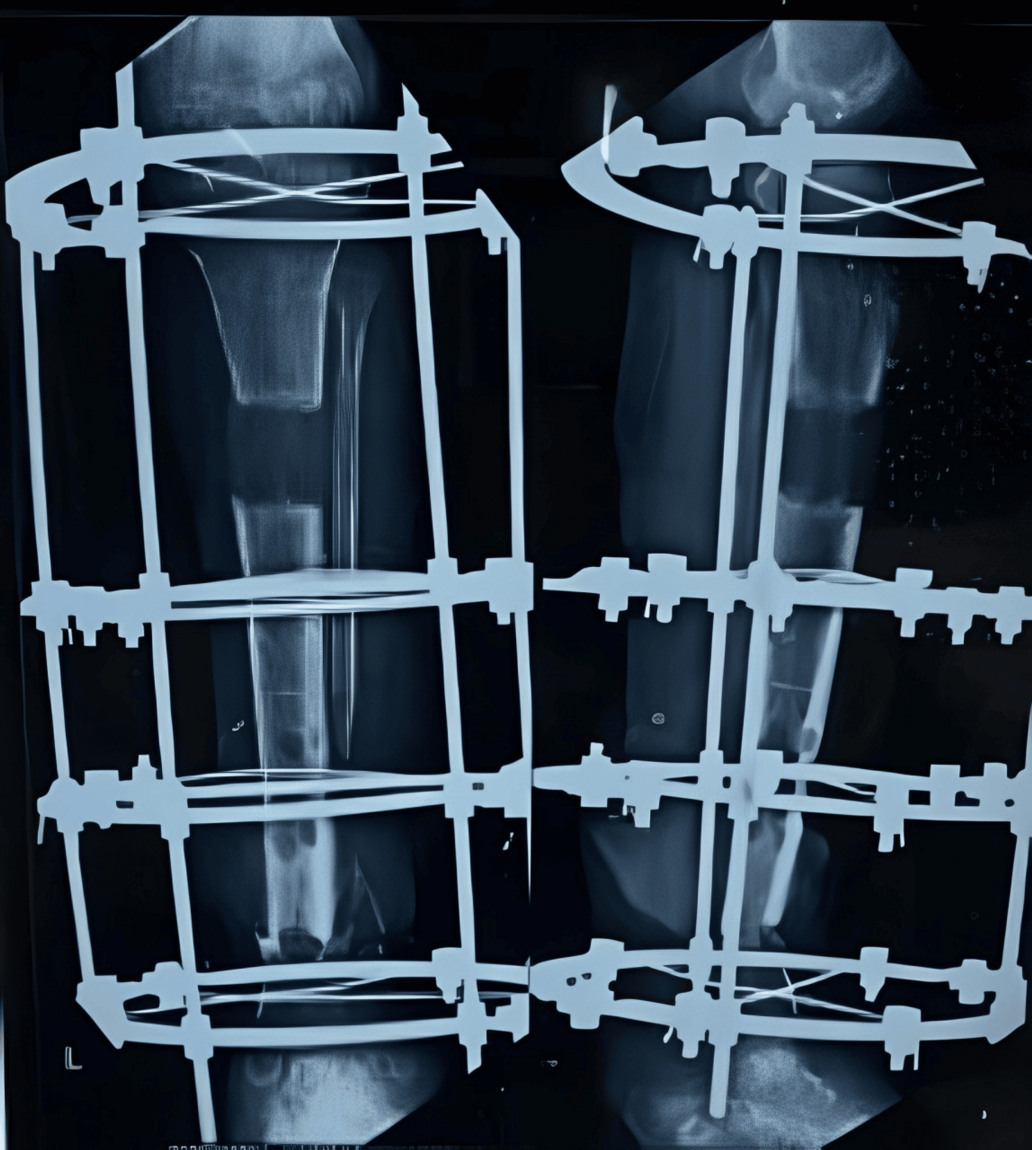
X-ray after three months of distraction
